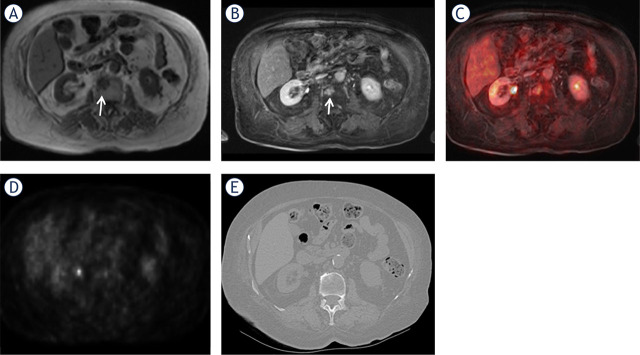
A 53-year-old woman with breast cancer. A metastasis can be observed in the L2 vertebral body (arrows) on the precontrast T1 weighted (W) image (A), contrast enhanced (CE) T1W image (B), and CE T1W VIBE-PET fused image (C). The AC PET axial image (D) does not show FDG uptake. CT yielded false-negative results (E). AC PET = attenuation-corrected raw data positron-emission tomography; FDG = fluorodeoxyglucose; VIBE = volume-interpolated breath-hold examination
